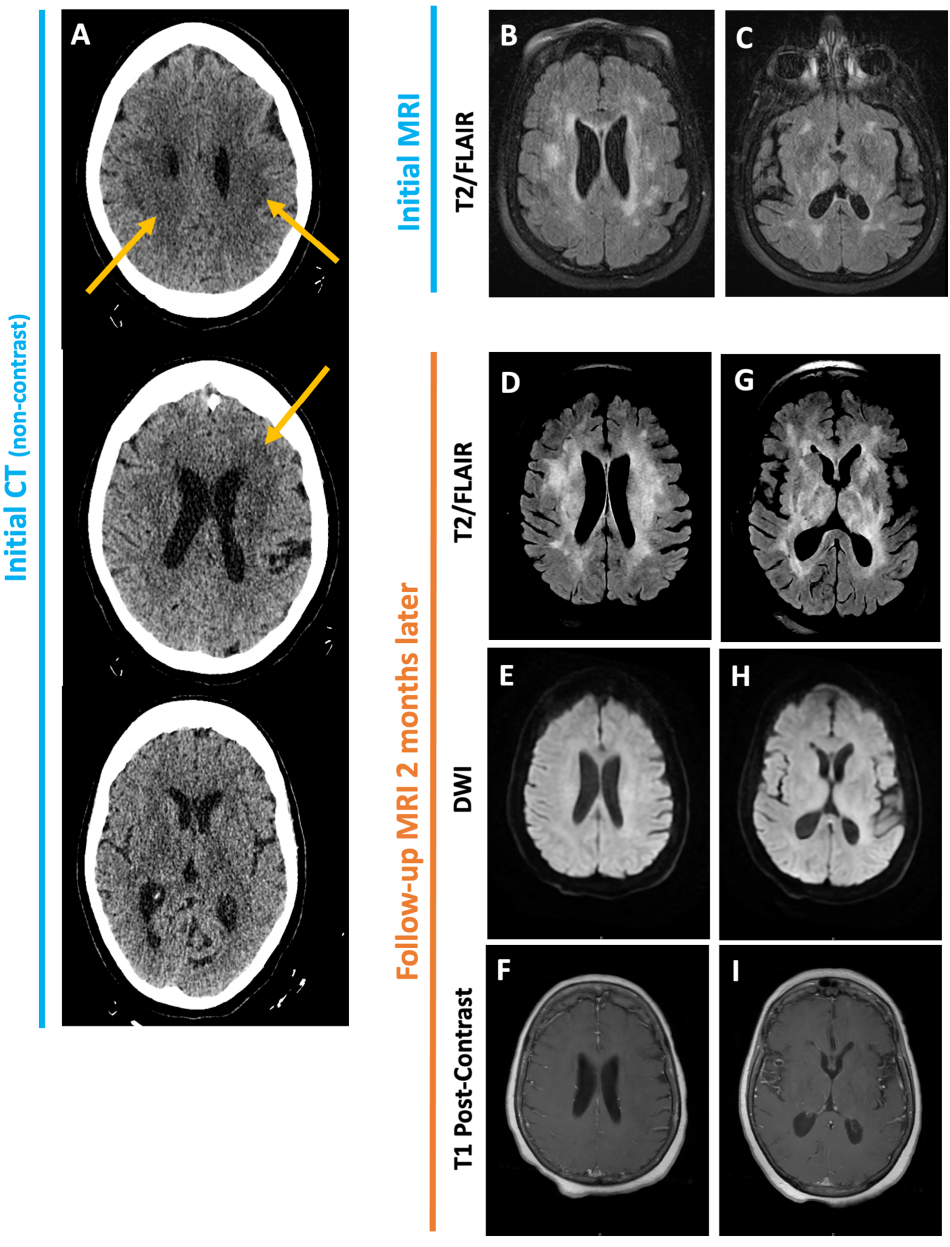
Initial non-contrast CT head (A) obtained for altered mental status shows ill-defined periventricular and subcortical white matter hypodensities. No evidence of intracranial hemorrhage, mass effect, or large territorial infarct. Initial MRI brain shows patchy T2/FLAIR hyperintensities in the periventricular and subcortical white matter as well as the bilateral basal ganglia (B, C). Follow-up MRI two months later reveals increased confluence of these lesions (D, G). No associated enhancement, diffusion restriction or mass effect is seen (E, F, H, I).
